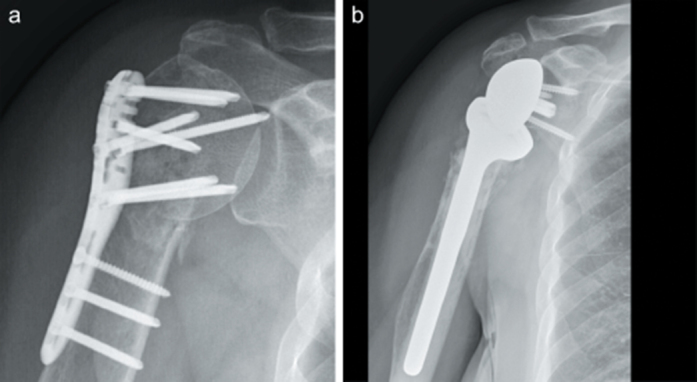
A 66-year-old male patient sustains shoulder pain that comes along with a crepitation and grinding sensation 1-month after ORIF with a locking plate for proximal humerus fracture. X-ray graphs reveal loss of reduction and screw penetration (a). The patient who has rheumatoid arthritis and osteoporosis was treated with a cemented RTSA after 1 year from index surgery (b).
